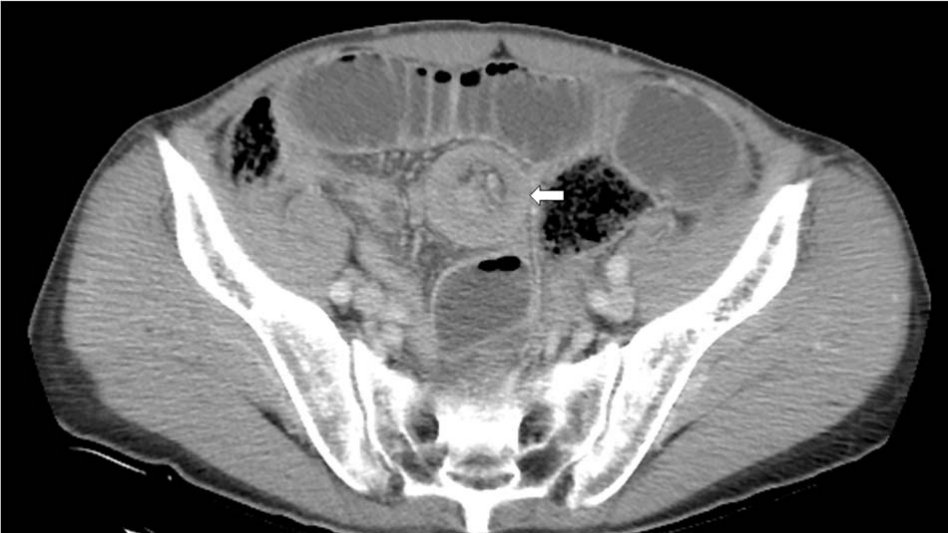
CT discloses a typical target sign over the small intestine (arrow).
